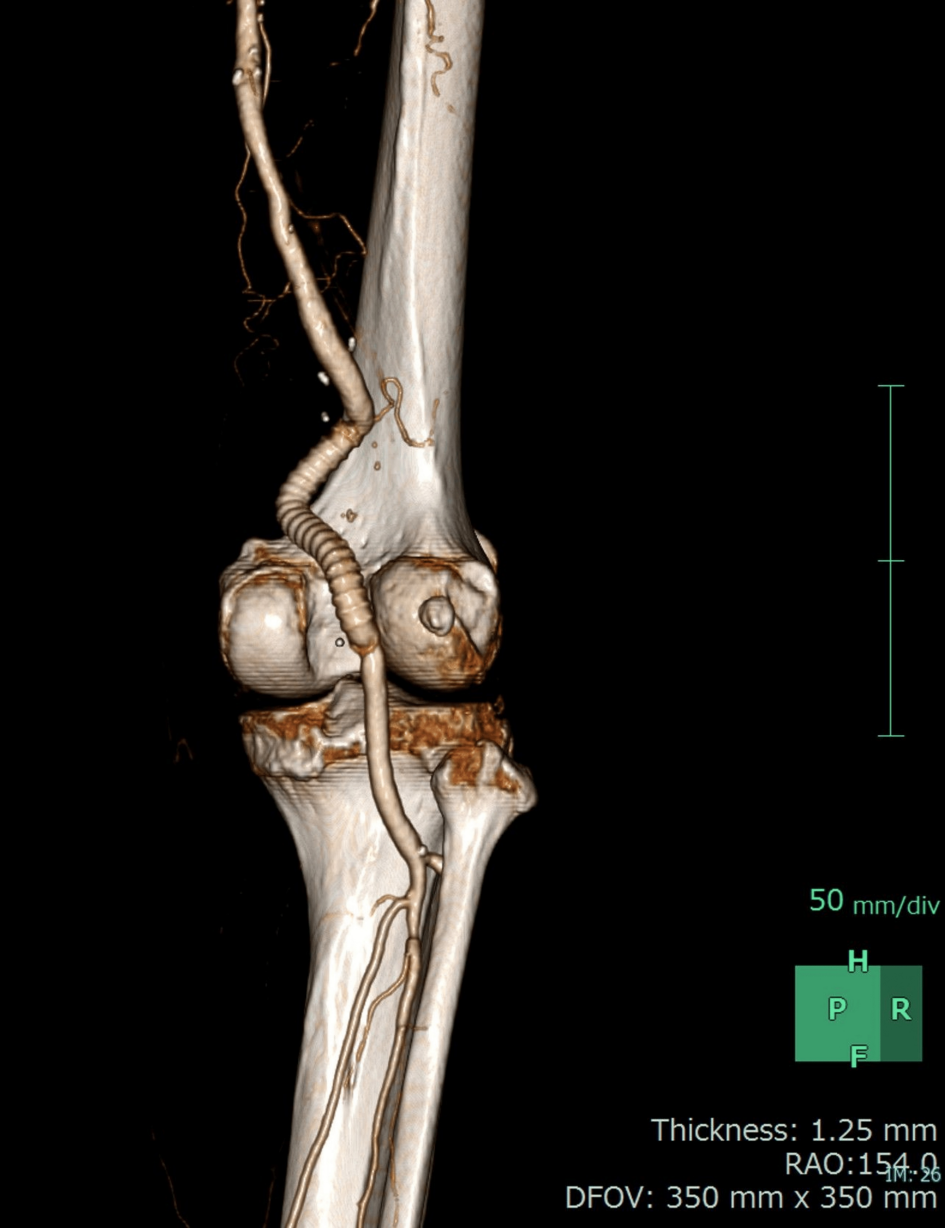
The postoperative three-dimensional computed tomography image showing the reconstruction using a prosthetic graft.
